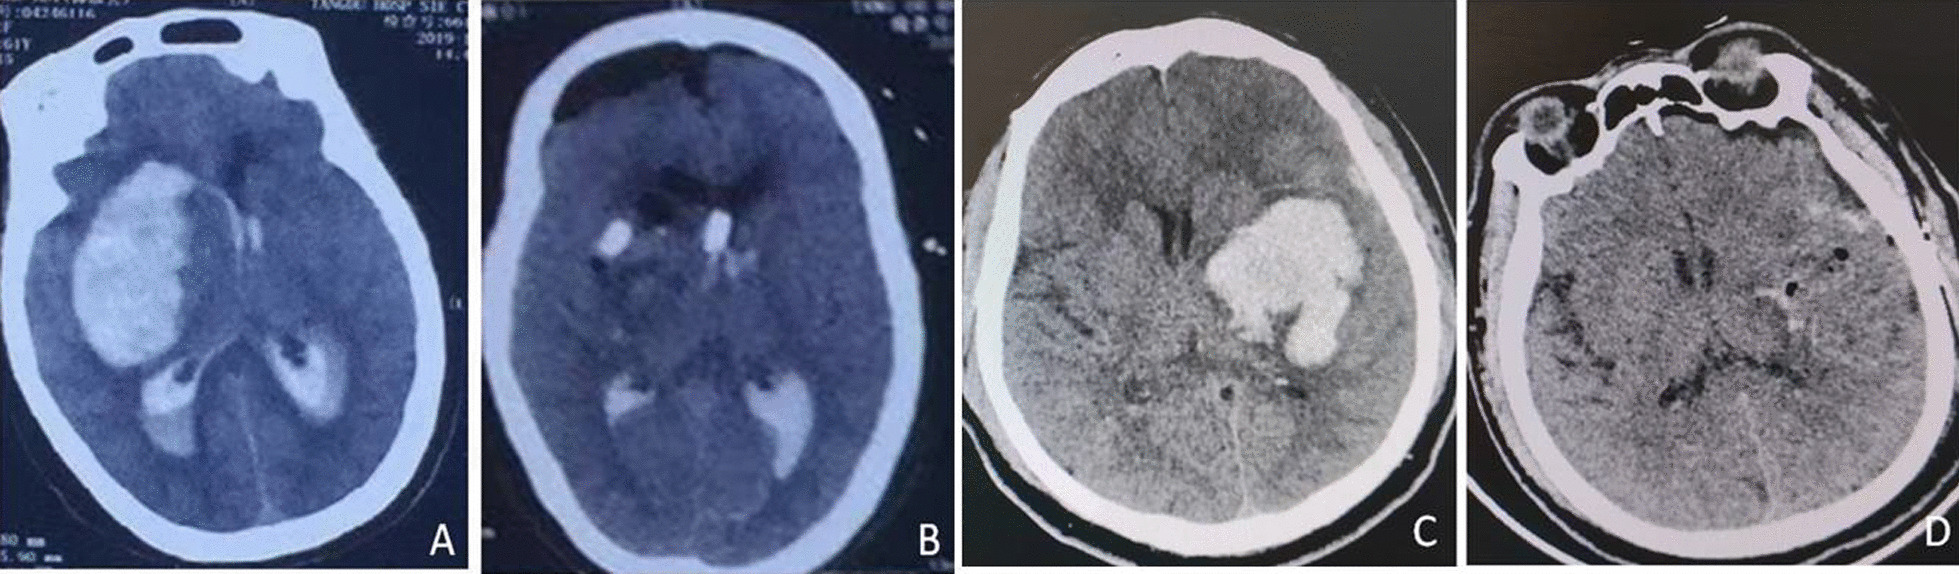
A CT images before Neuroendoscopic-assisted surgery; B CT images within 24 h after Neuroendoscopic-assisted surgery, Intraventricular hematoma wastreates with extracerebral drainage; C CTimages before mini-open caniotomy surgery; D CT images within 24 h after mini-open craniotomy
